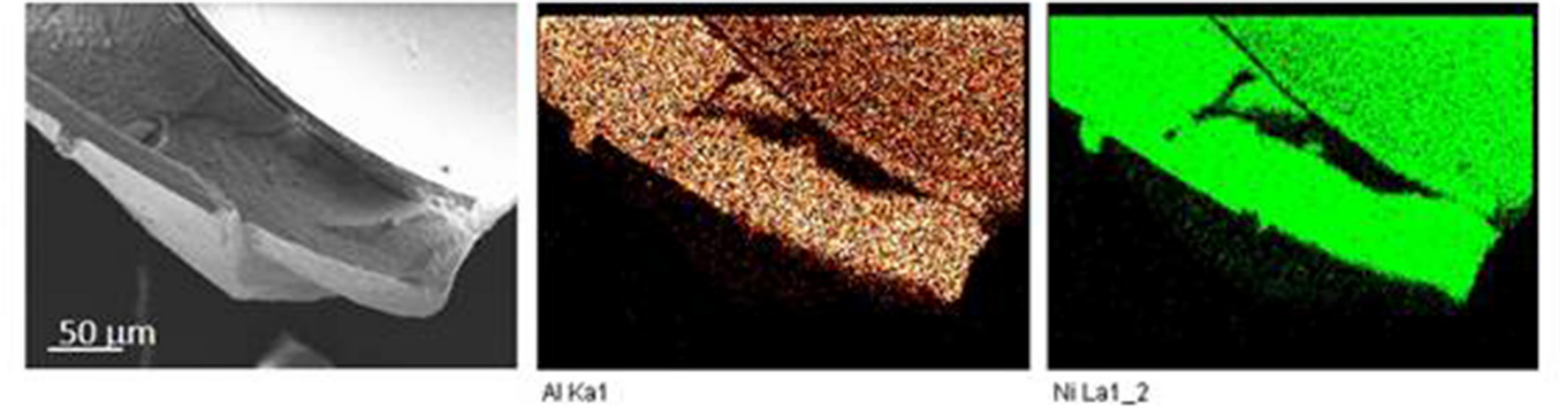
Energy dispersive X-ray (EDX) mapping analysis of NiAl@Alg-Ca.
